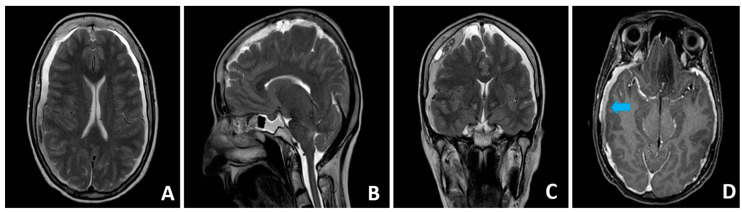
The MRI scan of the brain showed typical findings of intracranial hypotension. Axial and coronal T2-weighted images showing bilateral subdural fluid collections and reduction in size of the ventricular system (A,C). Sagittal T2-weighted image showing caudal descent of the brainstem and third ventricle, with minimal tonsillar herniation through the foramen magnum (B). Axial T1-weighted image after intravenous administration of gadolinium showing characteristic dural enhancement (D). Blue arrow: maximum thickness of subdural hematoma.
